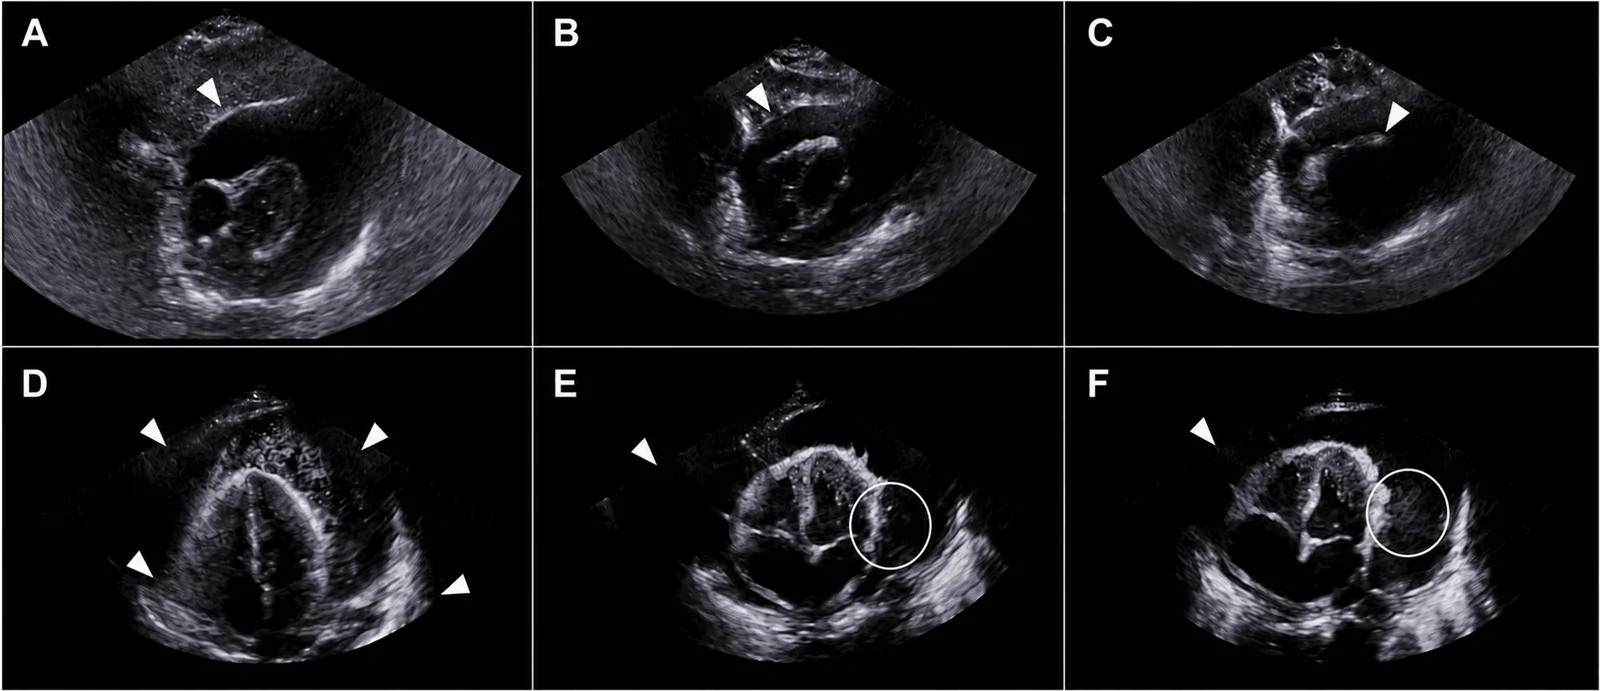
Transthoracic echocardiogram demonstrating a large pericardial effusion. Global left ventricular ejection fraction was approximately 55%. Diastology was indeterminate. Right ventricular cavity size appeared moderately dilated. Transthoracic echocardiography demonstrated a large circumferential pericardial effusion. Parasternal short-axis views (A-C) showed extensive pericardial fluid surrounding the left ventricle. Apical four-chamber views (D-F) confirmed the large circumferential effusion and demonstrated moderate right ventricular dilatation. Left ventricular size and systolic function were preserved, with an estimated ejection fraction of approximately 55% and no regional wall motion abnormalities. The inferior vena cava was dilated with less than 50% inspiratory collapse, and significant respiratory variation was present. Although these findings raised concern for evolving tamponade physiology, there was no echocardiographic evidence of right atrial or right ventricular collapse. A large pleural effusion was also noted.
